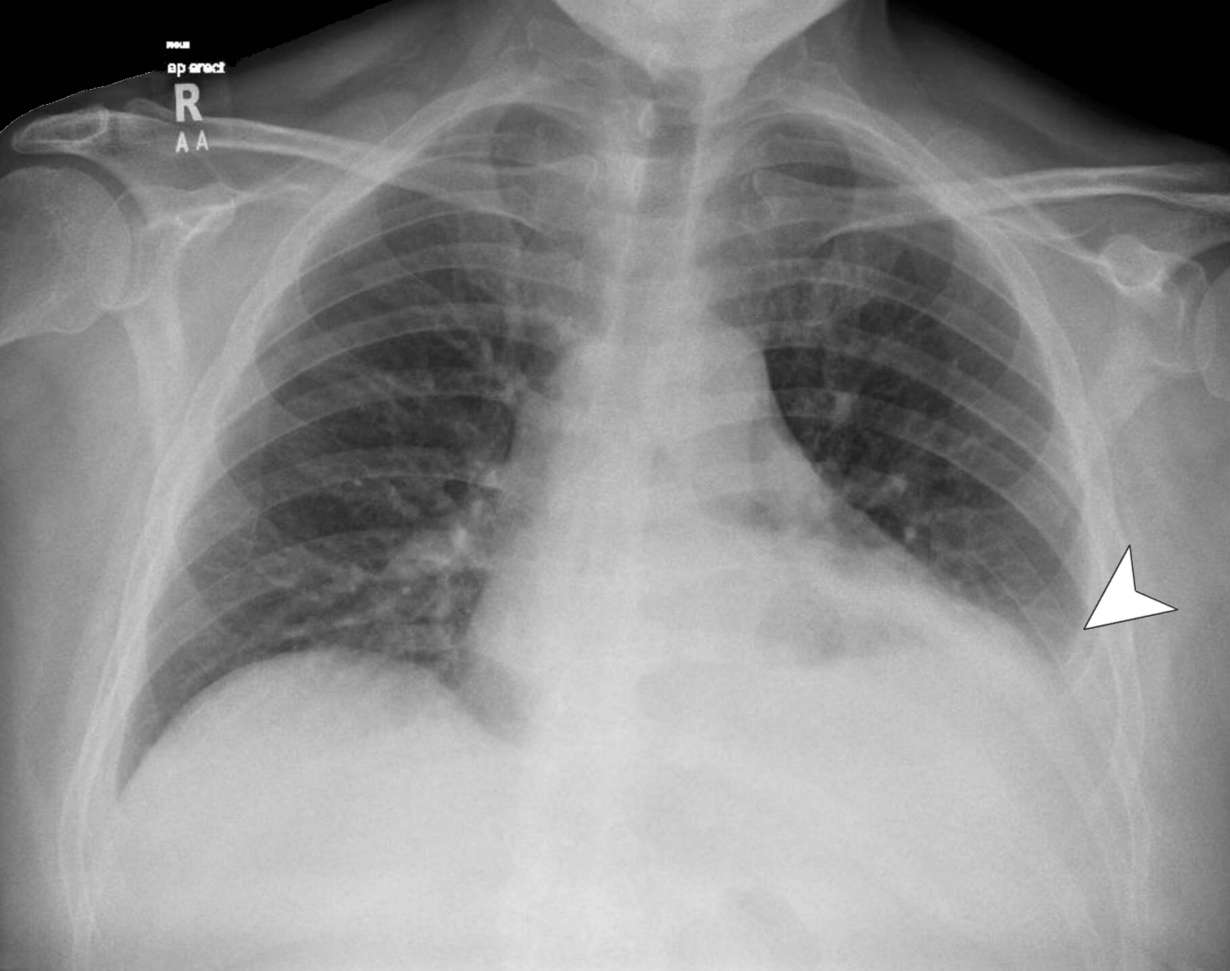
Chest X-ray showing left-sided pleural effusion
